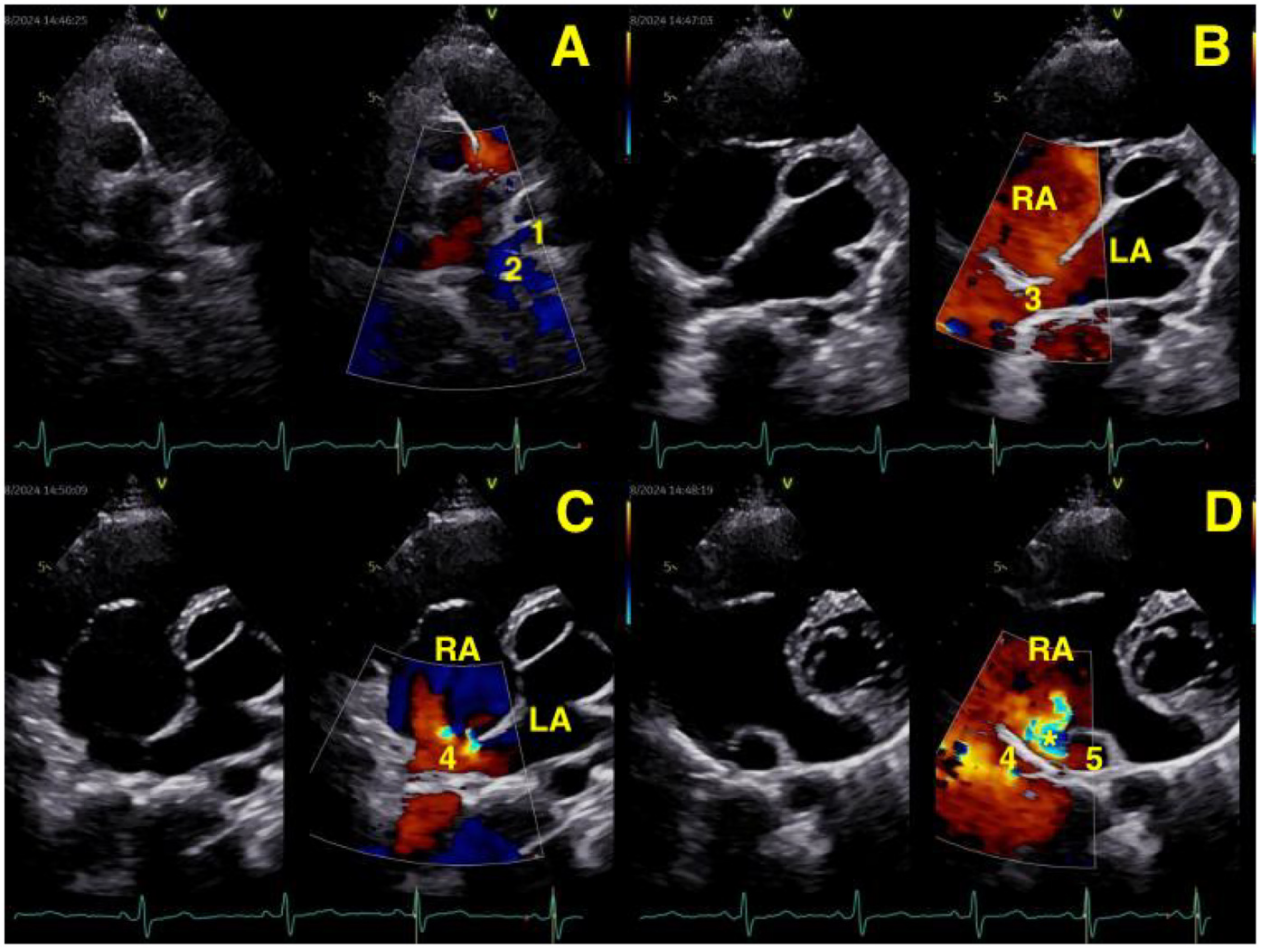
(A,B) Transthoracic echocardiography (TTE) showing the left lower pulmonary vein (1), the left upper pulmonary vein (2), and the right upper pulmonary vein (3) entering the left atrium. (C,D) TTE showed the right inferior pulmonary vein (4) draining into the left atrium, with Color Doppler revealing a bright, abnormal flow signal at this site. An orifice (*) representing an abnormal communication between the dilated the right lower pulmonary vein (4) and inferior vena cava (5) is visualized below the posterior wall of the right atrium, adjacent to the coronary sinus.
